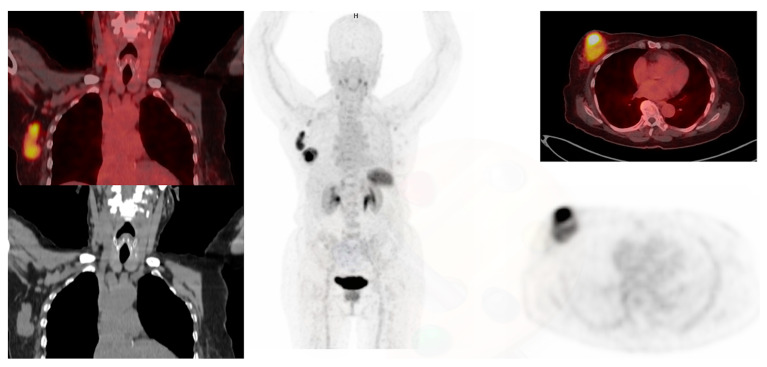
A 74-year-old female with triple-negative breast cancer underwent ^68^Ga-Pentixafor PET/CT. The images demonstrate inhomogeneous CXCR4 receptor expression in the primary lesion in the right breast as well as right axillary lymph nodes.
